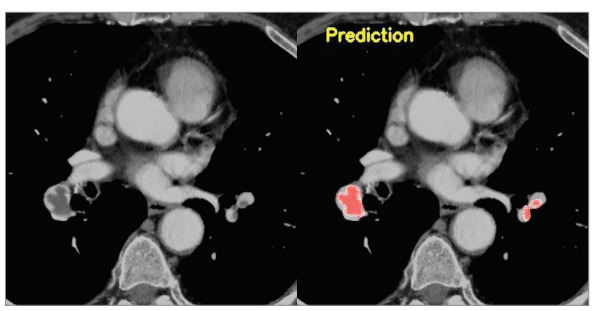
Computed tomographic pulmonary angiography examination revealed filling defects consistent with acute pulmonary embolism in the pulmonary arteries and segment branches of the lower
